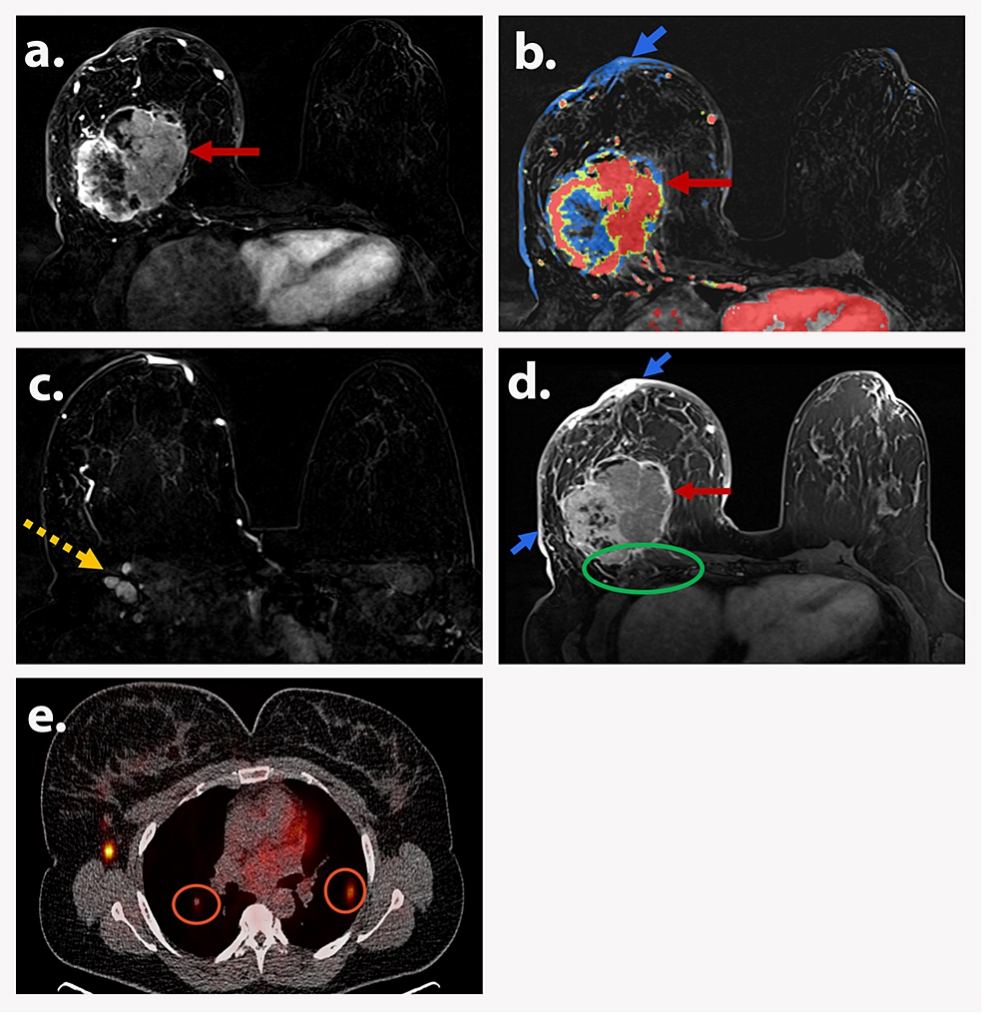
AJCC TNM Stage IV (T4cN1M1) A 46-year-old premenopausal woman presented to the emergency room with skin rash, swelling, and suspected abscess of the right breast. Axial T1-weighted subtraction DCE image (a) and corresponding color-coded map (b) show a 10.5 cm round, heterogeneously enhancing mass (red arrows) in the right breast with mixed kinetics. Evidence of diffuse skin thickening and enhancement (short blue arrow) is also noted. Axial T1-weighted subtraction DCE image (c) shows level 1 right axillary lymphadenopathy (dotted yellow arrow). Axial postcontrast T1-weighted image (d) shows diffuse right breast skin thickening (short blue arrows) and the mass (red arrow) abutting the pectoralis major muscle with obliteration of the fat plane and enhancement of the muscle (green circle). Axial PET/CT image shows bilateral FDG-avid pulmonary metastasis (orange circles). Biopsy depicted invasive carcinoma. Findings are consistent with AJCC TNM stage IV (T4cN1M1). AJCC: American Joint Committee on Cancer; TNM: tumor, node, and metastasis; DCE: dynamic contrast-enhanced; PET/CT: positron emission tomography/computed tomography; FDG: fluorodeoxyglucose.
